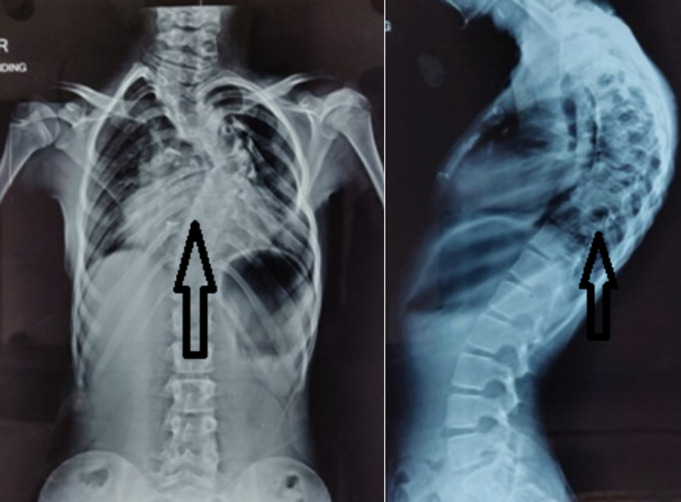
pre-op - X-Ray of the whole spine; (A) standing view shows thoracic curve, uev-d5, lev-d11, apex-d7, cobb- 42 degree; (B) supine side bending view shows proximal thoracic curve, uev- d1, lev -d5, apex-d2, Cobb -40 degree
